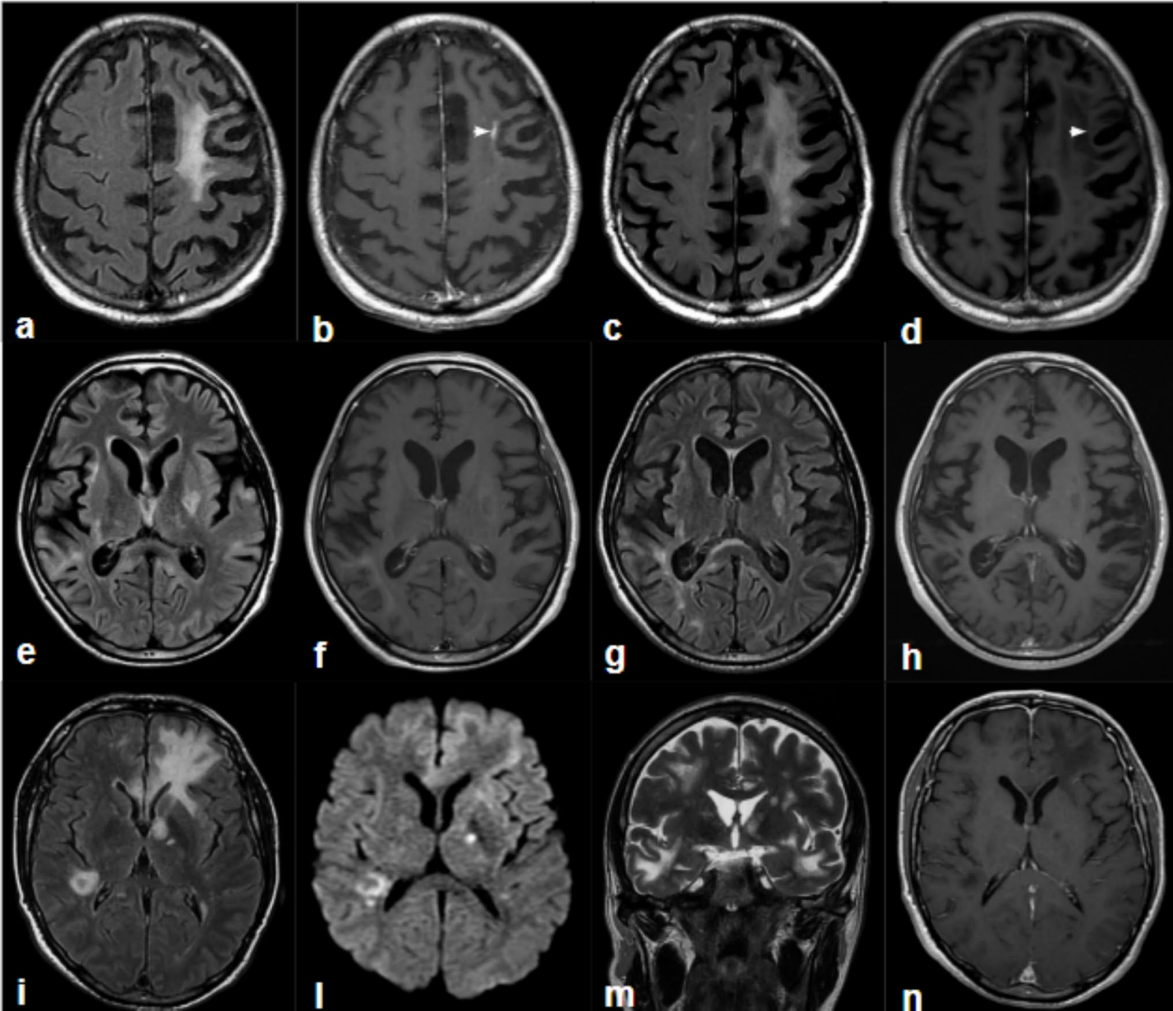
MRI imaging. Patient 1 (first Row, a-d): FLAIR (a) and T1-weighted post-contrast images (b) from the first MRI exam, and the corresponding FLAIR (c) and T1-weighted post-contrast images (d) after three years. The initial exam shows a FLAIR hyperintensity in the white matter of the left frontal lobe, involving the subcortical U fibers, with no mass effect and faint marginal enhancement (arrow in b), consistent with typical PML findings. Over time, the FLAIR lesion remains stable, while contrast enhancement resolves (arrow in d). Patient 3 (second Row, e-h): FLAIR (e) and T1-weighted post-contrast images from the first MRI exam, and the corresponding FLAIR (g) and T1-weighted post-contrast images (h) after 12 months. The initial FLAIR images reveal bilateral, patchy, ill-defined cortical and subcortical hyperintense lesions without mass effect; some lesions exhibit faint marginal enhancement. These imaging findings are atypical for PML. The follow-up MRI shows a reduction in lesion size, persistent FLAIR hyperintensities, and evidence of ventricular and sulcal expansion. Patient 4 (third row, i-n): Single MRI exam with FLAIR (i), DWI (l), coronal T2 (m), and T1-weighted post-contrast (n) images. The MRI demonstrates multiple FLAIR hyperintense lesions involving the white matter of multiple bilateral lobes, the splenium, left internal capsule, and nucleus pallidus. DWI reveals variable signal intensities: lesions in the left frontal and right periventricular regions show a low-signal core surrounded by a high-signal rim, with a punctate high signal in the globus pallidus and intermediate-to-low signal in the corpus callosum. Coronal T2 imaging shows a slight mass effect on the left ventricle, which appears reduced in size compared to the contralateral side. No contrast enhancement is observed
